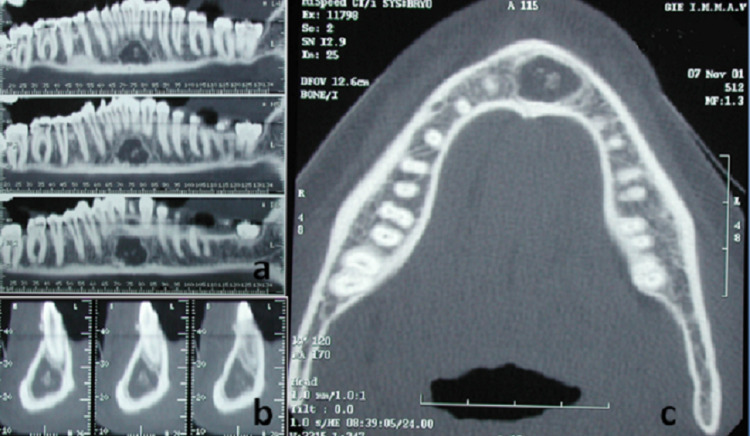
CT scan of panoramic reconstruction (a), cross-sectional (b), and axial (c) cuts showing a well-defined unilocular hypodense image centered by small round radiopacities located in the mandibular symphysis region
